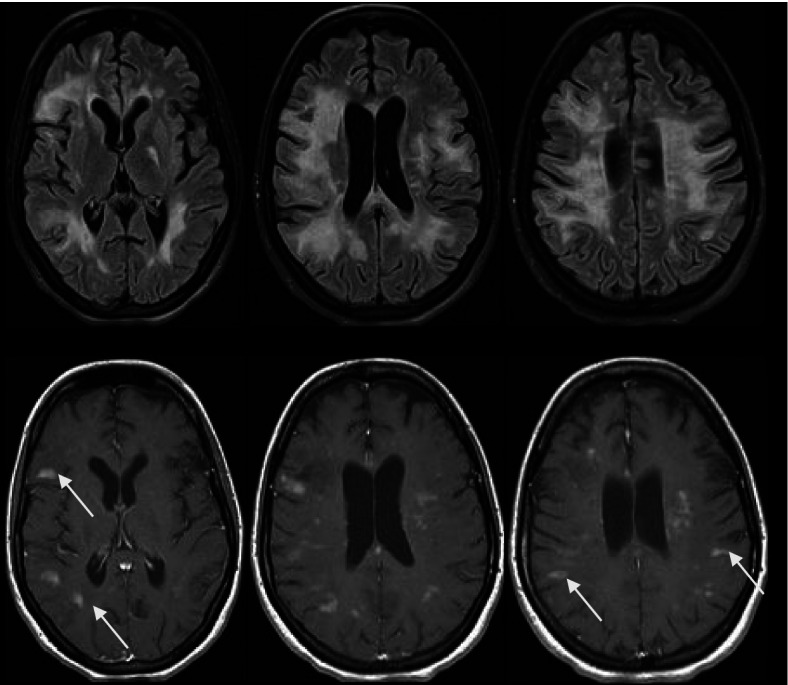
Another follow-up MRI of the patient (2020.11.24). A-C axial T2W FLAIR showed decreasing lesions and diminishing mass effect as well as new hyperintense lesions; D-F axial T1W with contrast revealed gadolinium enhancement in new lesions (arrows)
